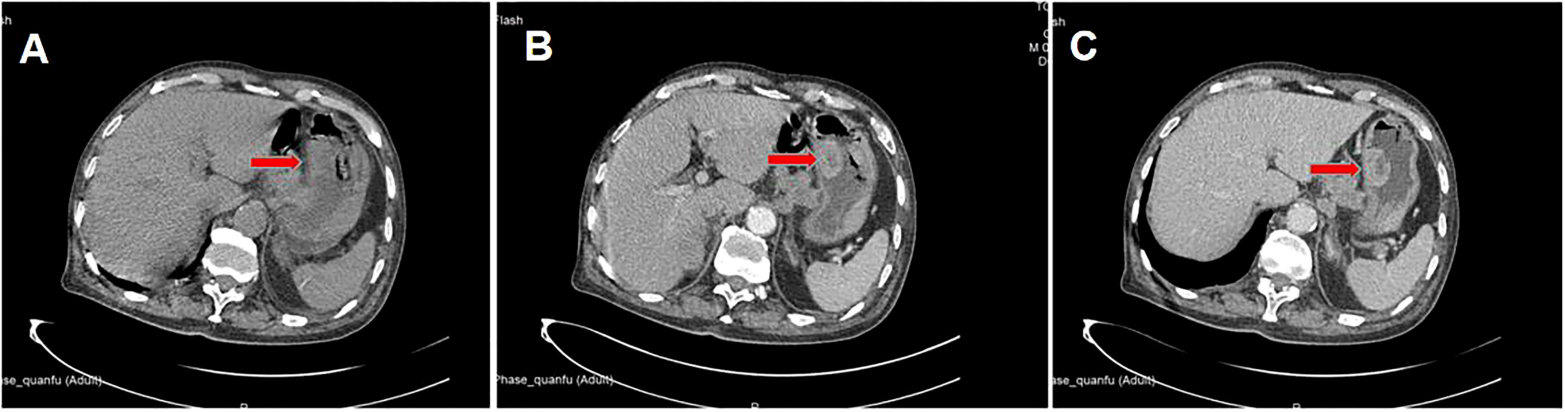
Location of the stomach tumor in abdominal enhanced CT. (A) Plain scan; (B) arterial phase; (C) venous phase.
